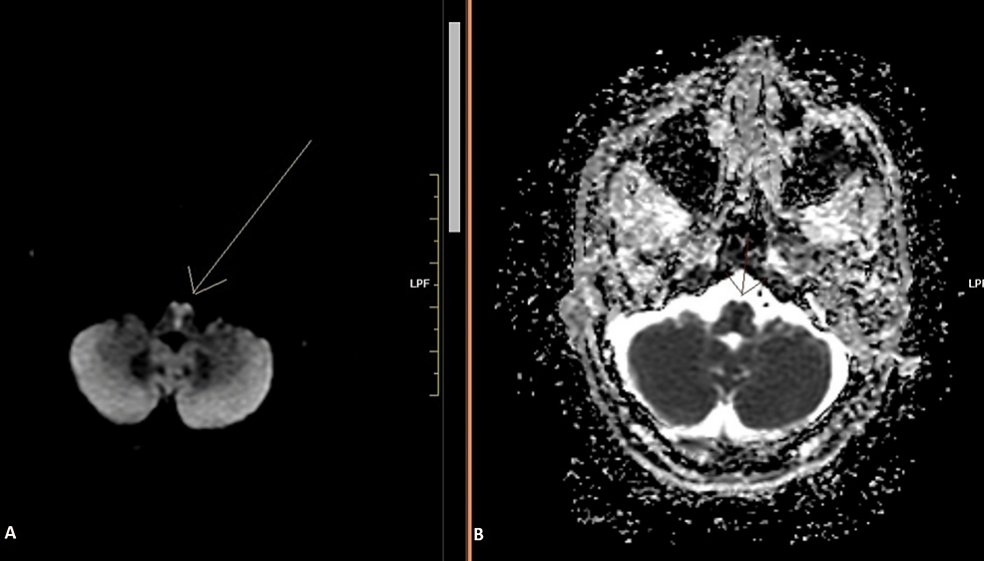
(A) A T2-weighted image showing the characteristic heart-shaped hyperintensity/airpod sign in the medulla, contrasted with (B) a corresponding drop in the apparent diffusion coefficient
